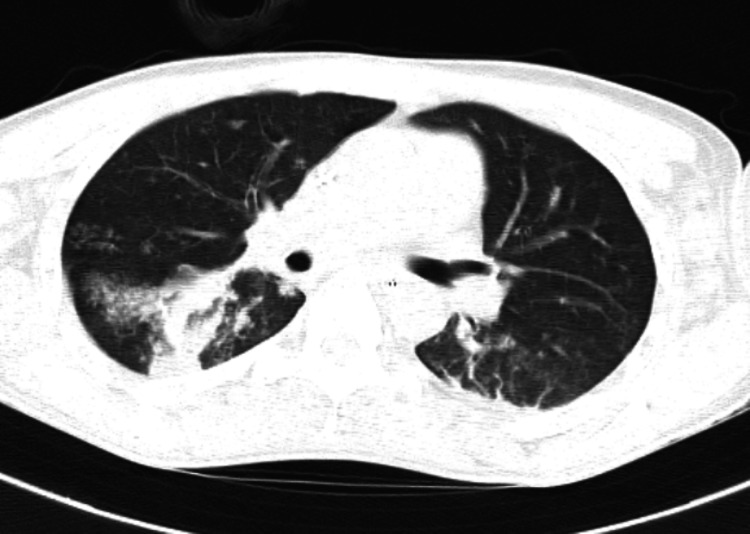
HRCT thorax shows bilateral tree in bud appearance. HRCT- High-resolution computed tomography
